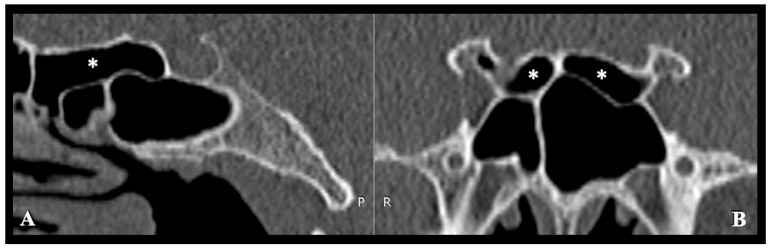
Computed tomography (CT) images demonstrate the presence and anatomical relationships of Onodi cells. (A) Sagittal CT image showing a unilateral Onodi cell (single asterisks, *) extending posteriorly and superolaterally to the sphenoid sinus (SS). (B) Coronal CT image revealing bilateral Onodi cells (single asterisks, *) positioned superior to the SS and closely related to the optic nerve canal. The Onodi cell, also known as the sphenoethmoidal cell, represents the most posterior ethmoidal air cell, which may extend into the sphenoid bone and create a potential risk for optic nerve or internal carotid artery exposure during endoscopic sinus or skull base procedures.
